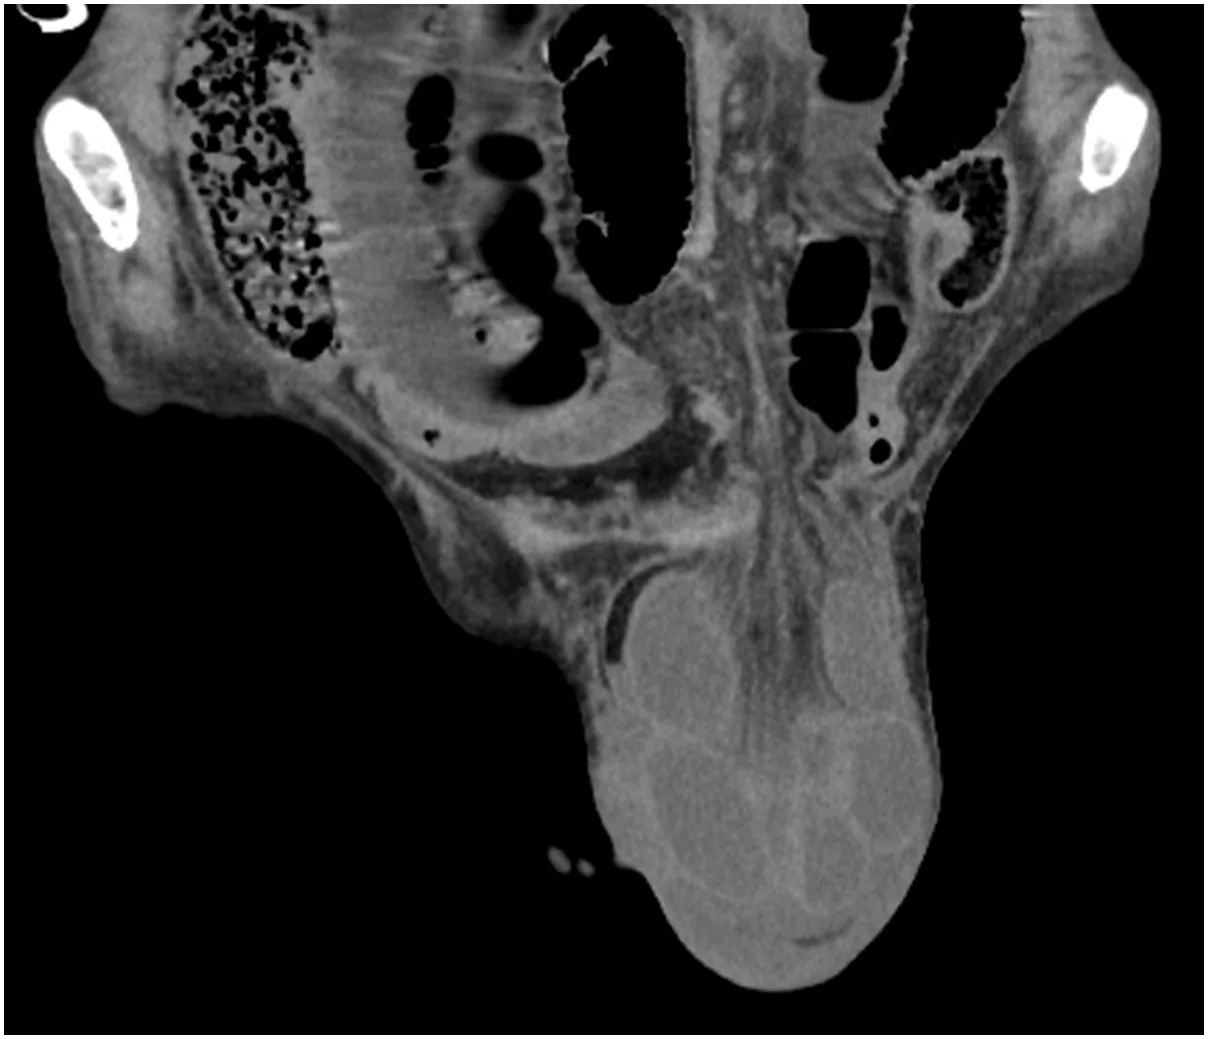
Pre-reduction CT imaging. Pre-reduction CT showing an incarcerated left inguinal hernia containing small bowel.
